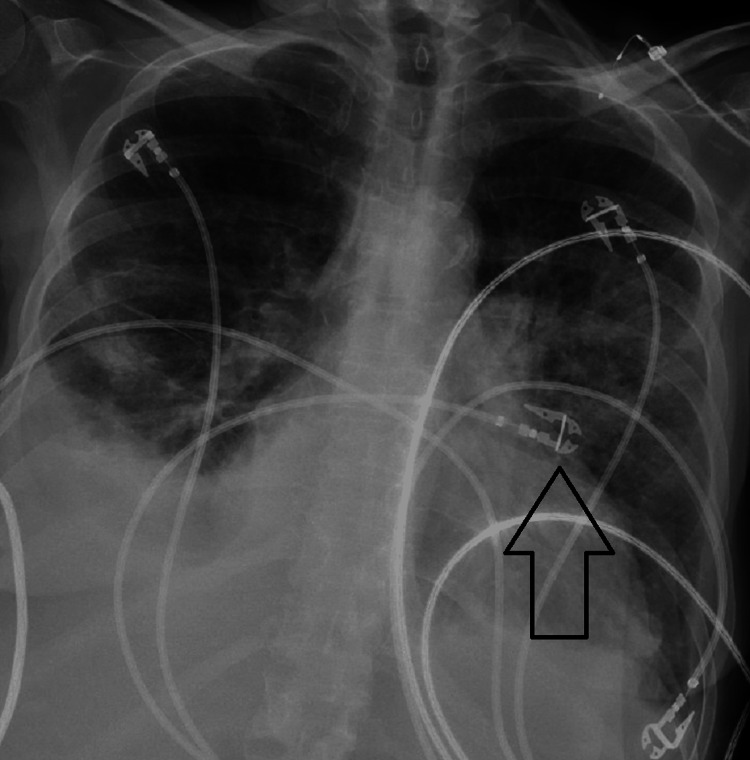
Follow-up chest x-ray demonstrating interval improvement of pulmonary edema, especially in the left lung (arrow), after multiple sessions of diuresis
